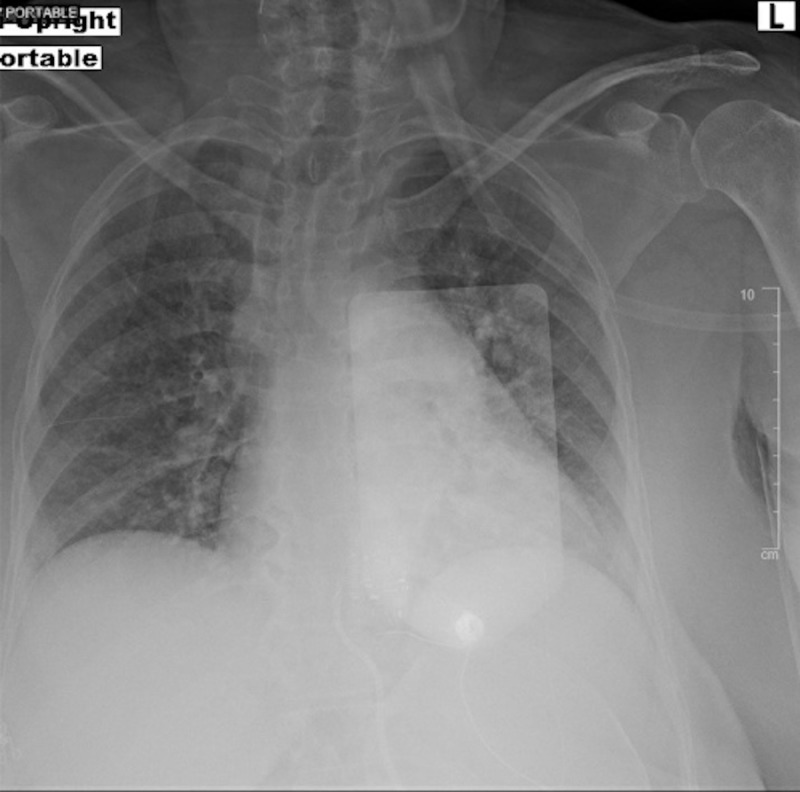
Portable chest X-ray on presentation showing diffuse, hazy, ground-glass airspace disease
